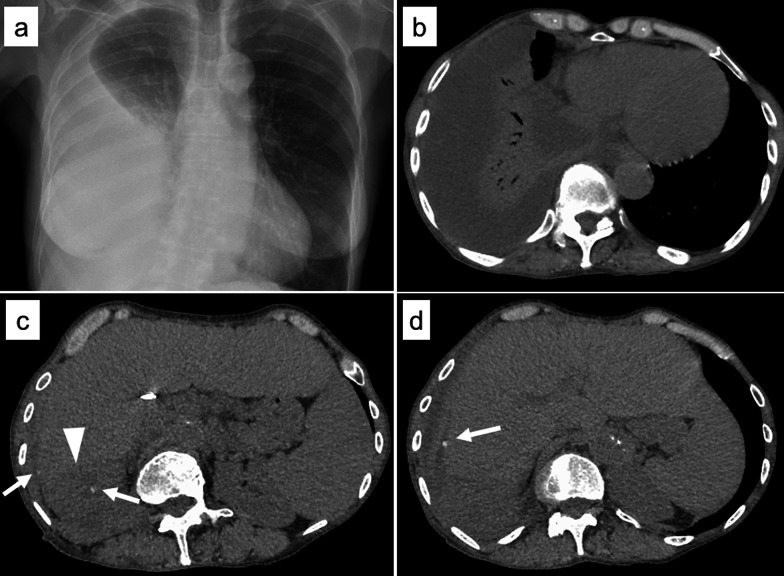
Chest radiography and CT at the time of pleural empyema diagnosis. a Chest radiography reveals a large amount of right pleural effusion. b Chest CT shows a large amount of right pleural effusion with passive atelectasis. c, d Abdominal CT shows perihepatic fluid collection (arrowhead) and multiple small radiopaque density masses (arrow)
